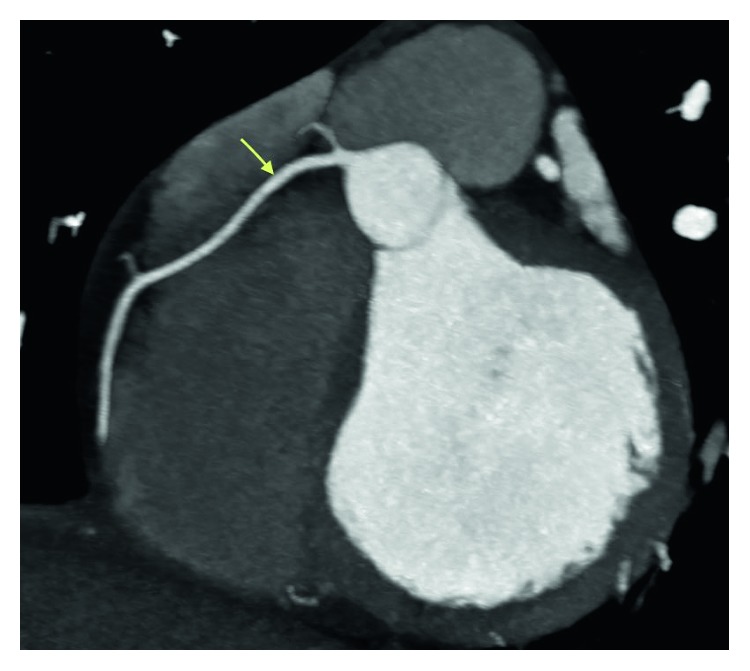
Coronary CT angiogram image revealing the right coronary artery arises normally from the right coronary cusp and is dominant without any evidence of coronary artery disease.
